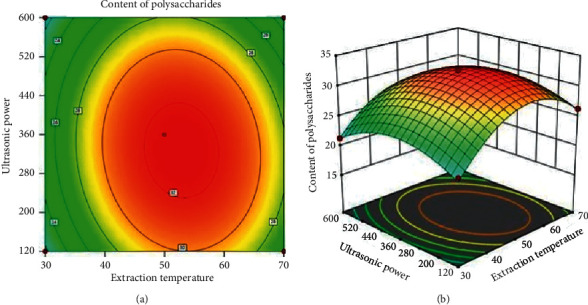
Simultaneous effect of extraction temperature and ultrasonic power on the content of polysaccharides.
